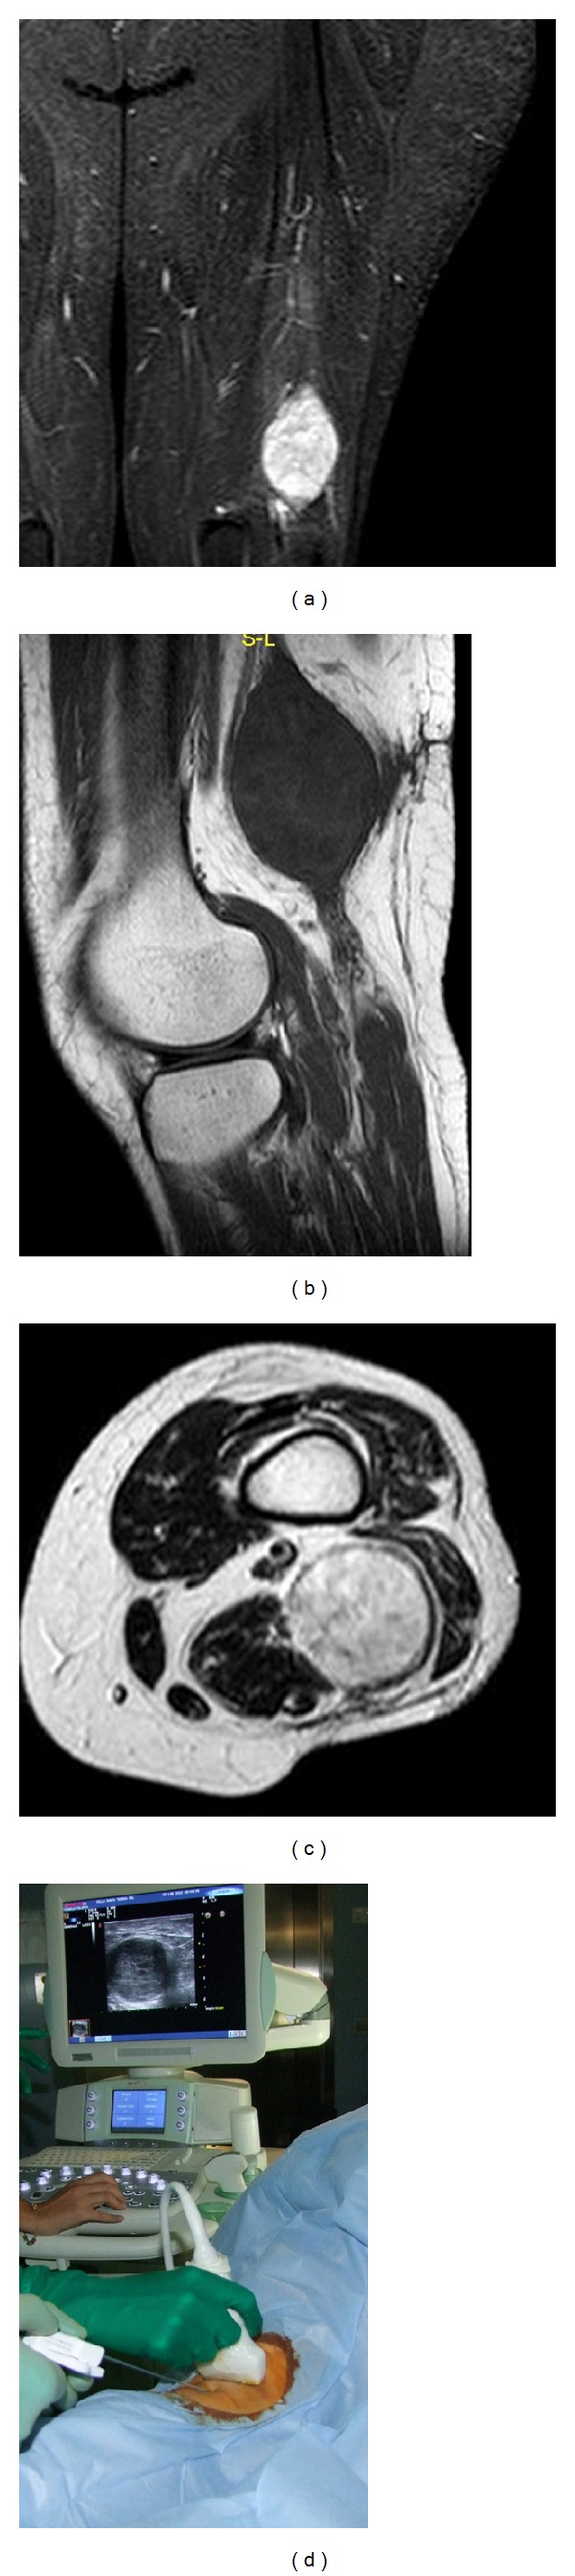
Coronal MRI STIR (a), sagittal MRI T2 (b), and axial MRI T1 (c) scans of the left thigh show homogeneous intratumoral hypointensive signal on T1 and hyperintensive signal on T2. A 14-gauge ultrasound-guided soft tissue percutaneous biopsy (d) of the left tight. Diagnosis: schwannoma.
